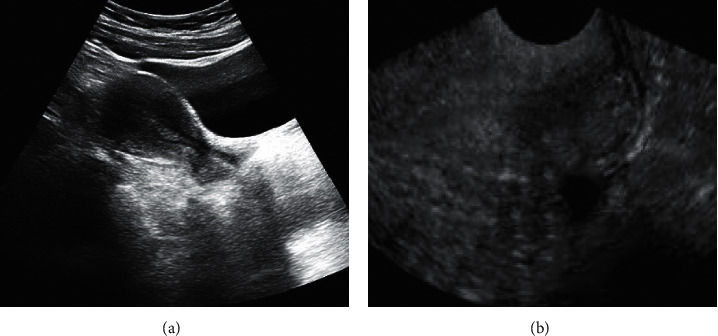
(a): Normal uterine cavity by transabdominal ultrasound; (b) normal uterine cavity by transvaginal ultrasound.
